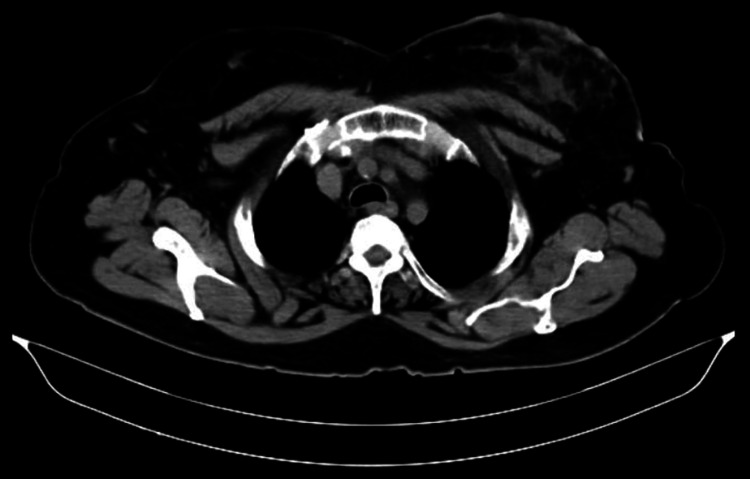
A contrast-enhanced CT scan of the abdomen and pelvis was performed, demonstrating an irregular non-enhancing lesion in the left breast measuring 8.3 x 5.3 x 3.2 cm.
